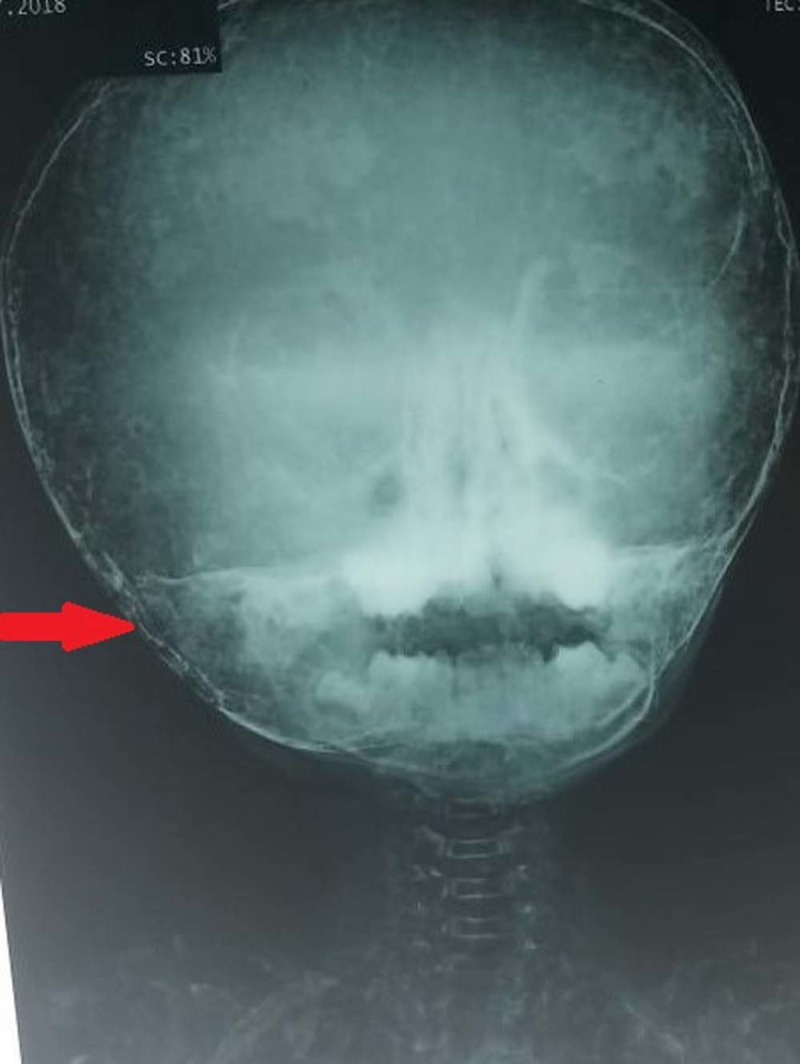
Skull X-ray showing thinning of the skull with multiple ossification centres.
